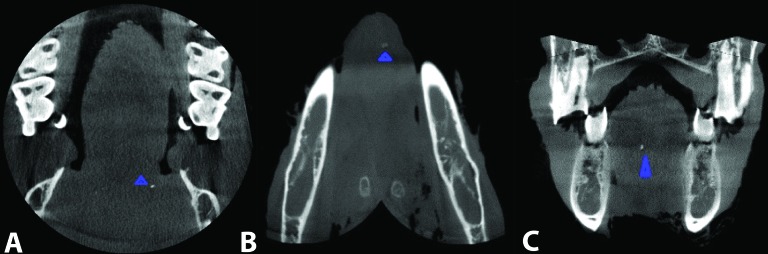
NewTom VG Cone Beam CT was used, with cone x-ray beam and 1920 × 1536-pixel flat panel detector, 15 × 15 cm detector size, 360 degree rotation, 18 s scan time and 120 kVp. The slices were prepared at kVp = 110 at a scan time of 18s and a minimum thickness of one millimeter. Initial and final reconstruction was performed using NNT Viewer software version 2.17 (Figure 2).
